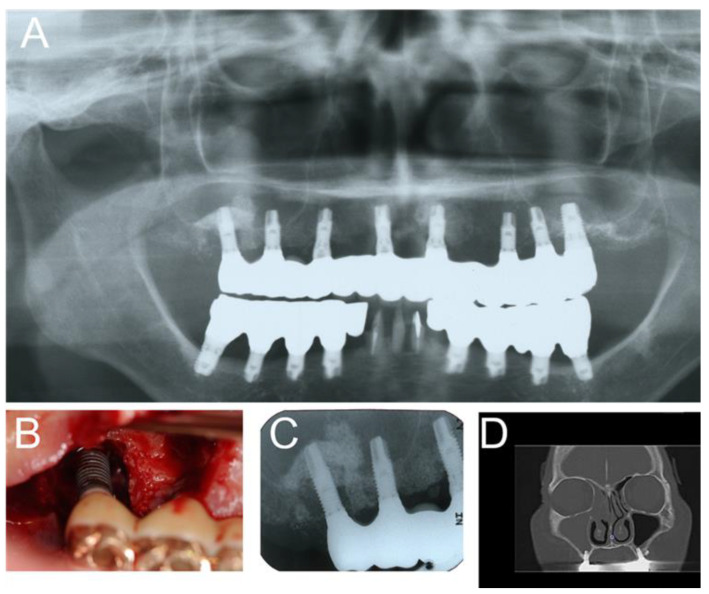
Severe peri-implantitis in the upper right molar region was observed 10 years after implant therapy with sinus augmentation. (A) Panoramic radiography; (B) oral photograph; (C) intraoral radiograph; (D) paranasal CT shows right rhinosinusitis. In this case, chronic maxillary sinusitis occurred through the pathway from peri-implantitis to the maxillary sinus for a long period. Therefore, FESS was performed (unpublished data).
